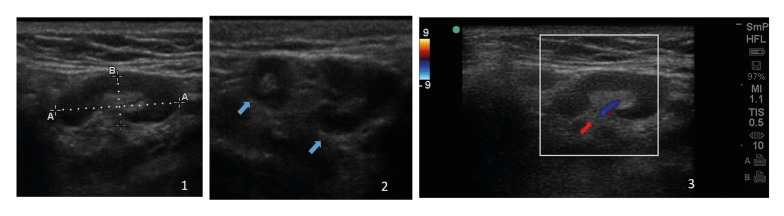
Ultrasonographic images (1: B-mode ultrasonographic appearance and size measurements of a submandibular lymph node, 2: Two different lymph nodes in a single image, blue arrows, and 3: Doppler ultrasound scanning of a submandibular lymph node with hilar vascularity, red arrow).
